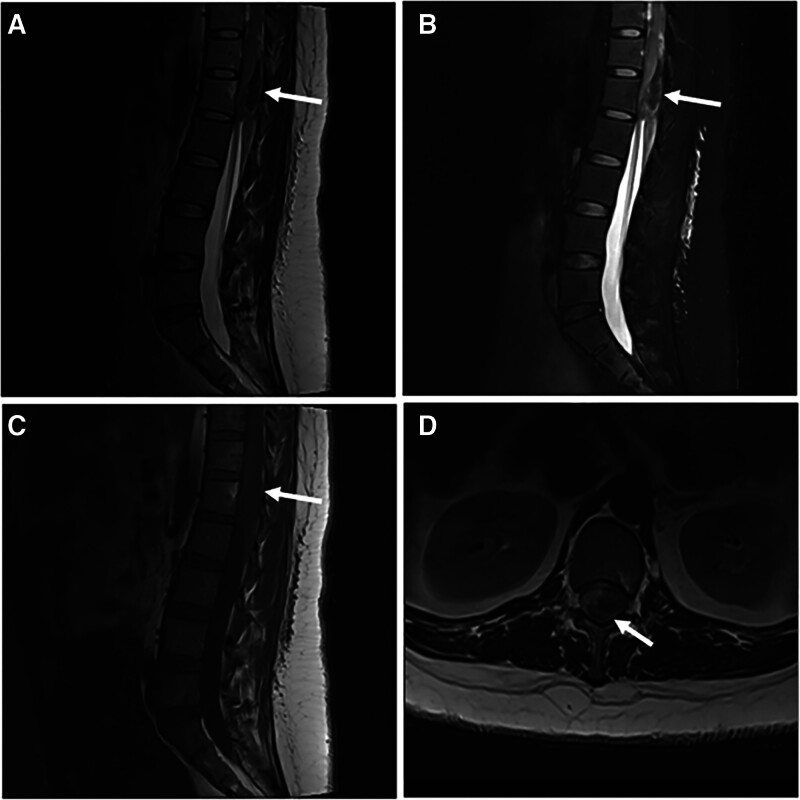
Preoperative thoracolumbar MRI scan prior to spinal cord decompression surgery. (A) Sagittal T2-weighted MRI image. (B) sagittal T2-weighted fat suppression MRI image. (C) Cagittal T1-weighted MRI image. (D) Axial T2-weighted MRI image at the level of L1. MRI revealed a heterogenous T1-isointense, T2-hyperintense lesion with mixed signals of about 8.6 × 1.6 cm at the level of T12–L2 in the subdural area, with poorly defined borders and uneven signals, and the lesion was poorly demarcated from the spinal cord cones, which were pushed out. The white arrows indicate space-occupying lesions. MRI = magnetic resonance imaging.
